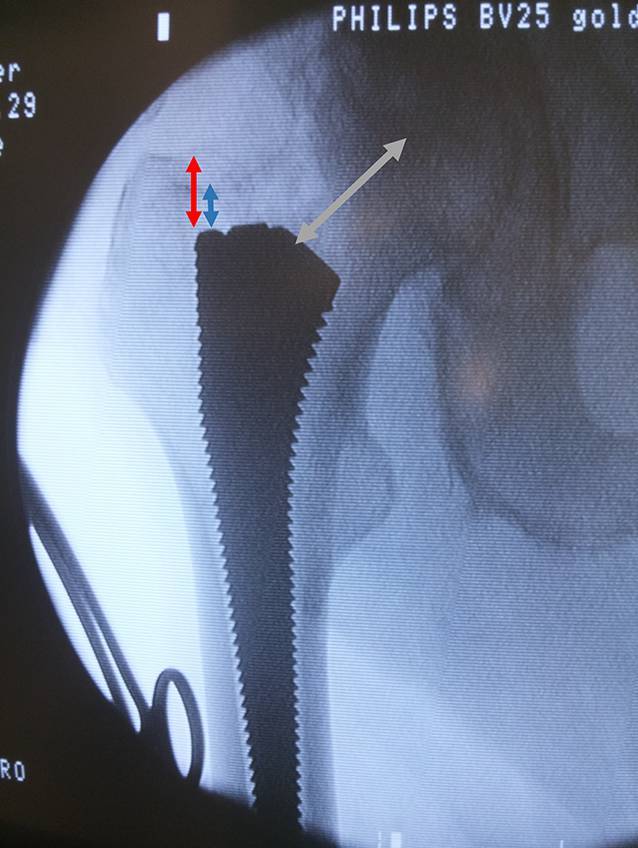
If desired compare intraoperative radiographic evaluation with the c‑arm and digital planning of the components
